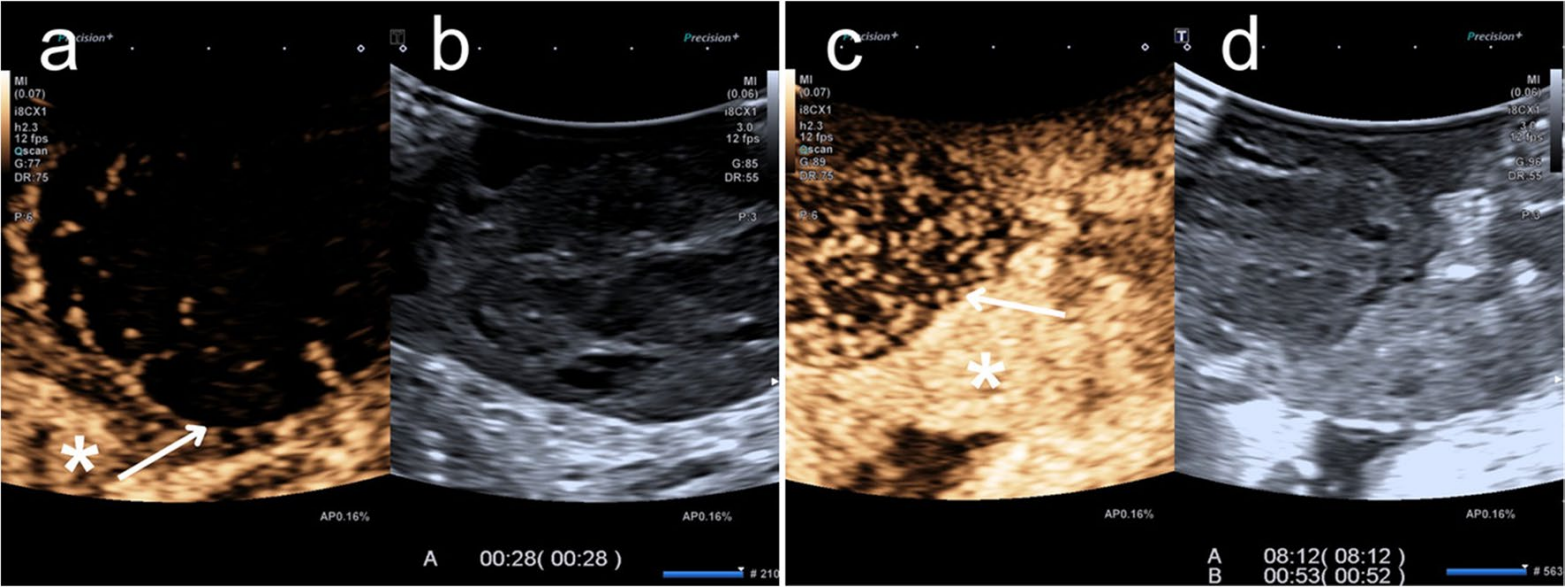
Intraoperative oblique contrast-enhanced ultrasound examination of the right kidney of a 5-year-old boy with reduced contrast agent uptake of the tumor tissue (*arrows*) compared to the healthy renal parenchyma (*asterisks*) in both the early (a and b) and late (c and d) contrast phase. a, c Contrast-enhanced sonographies. b, d Grayscale sonographies
